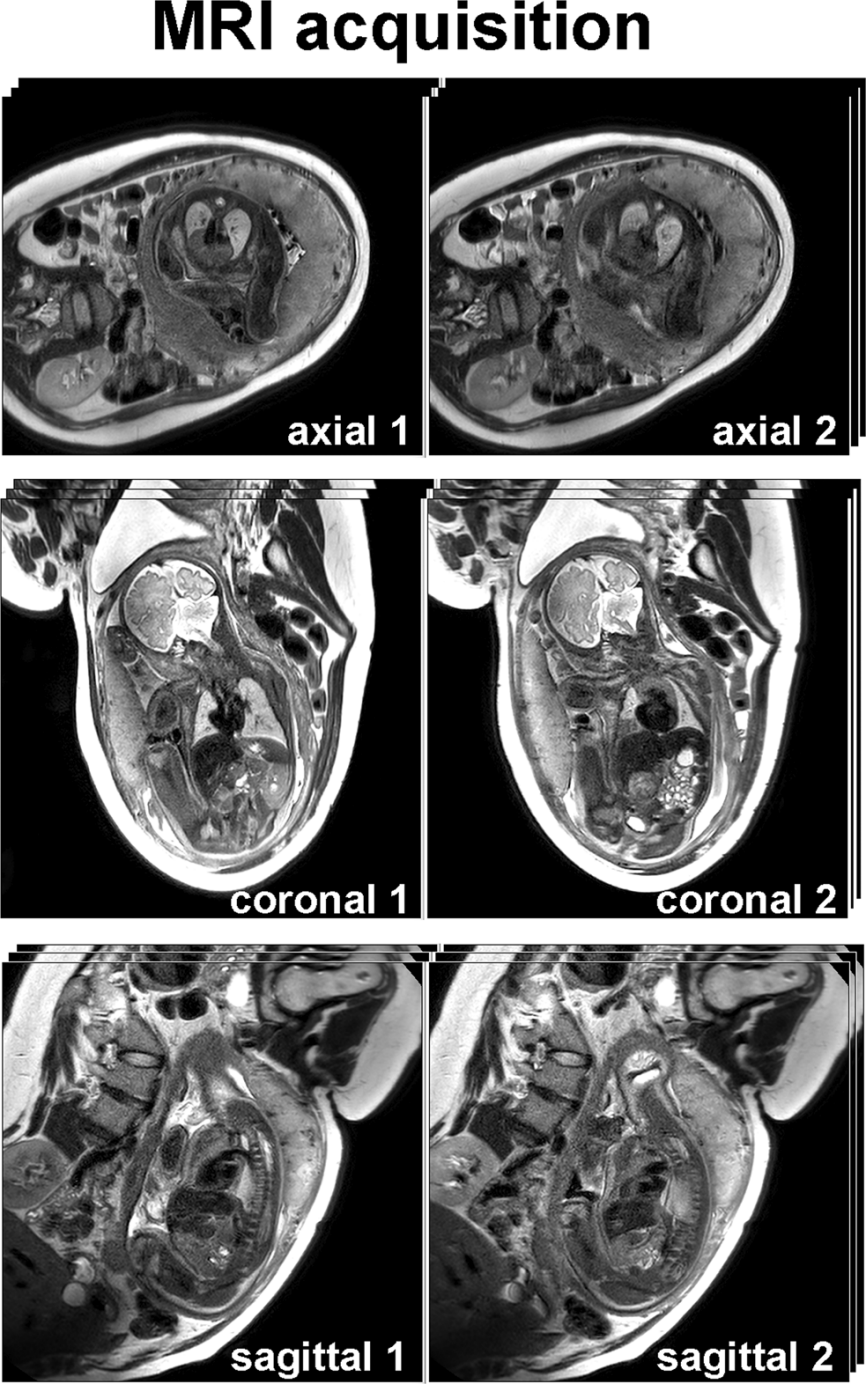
Presented are two axial, two coronal, and two sagittal stacks obtained in a T_2_‐weighted black blood acquisition at 3.0 T MRI. These acquisitions delineate the fetal position and its gross anatomy, and they can be used to reconstruct three‐dimensional volumes as presented in Figure 3.
